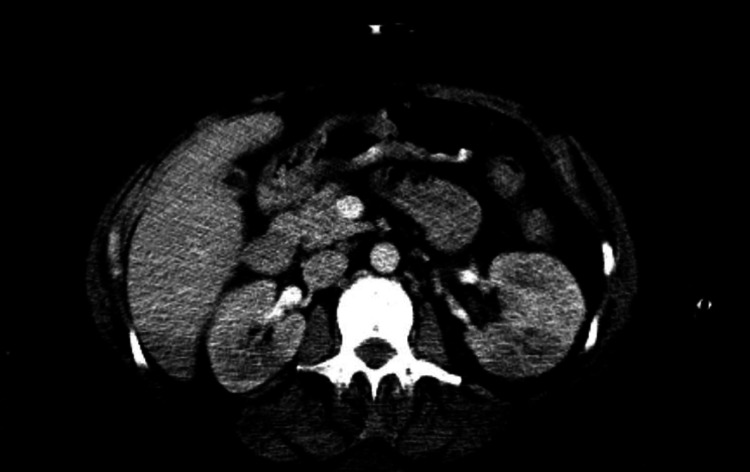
CT of the abdomen showing left and right renal infarcts.
